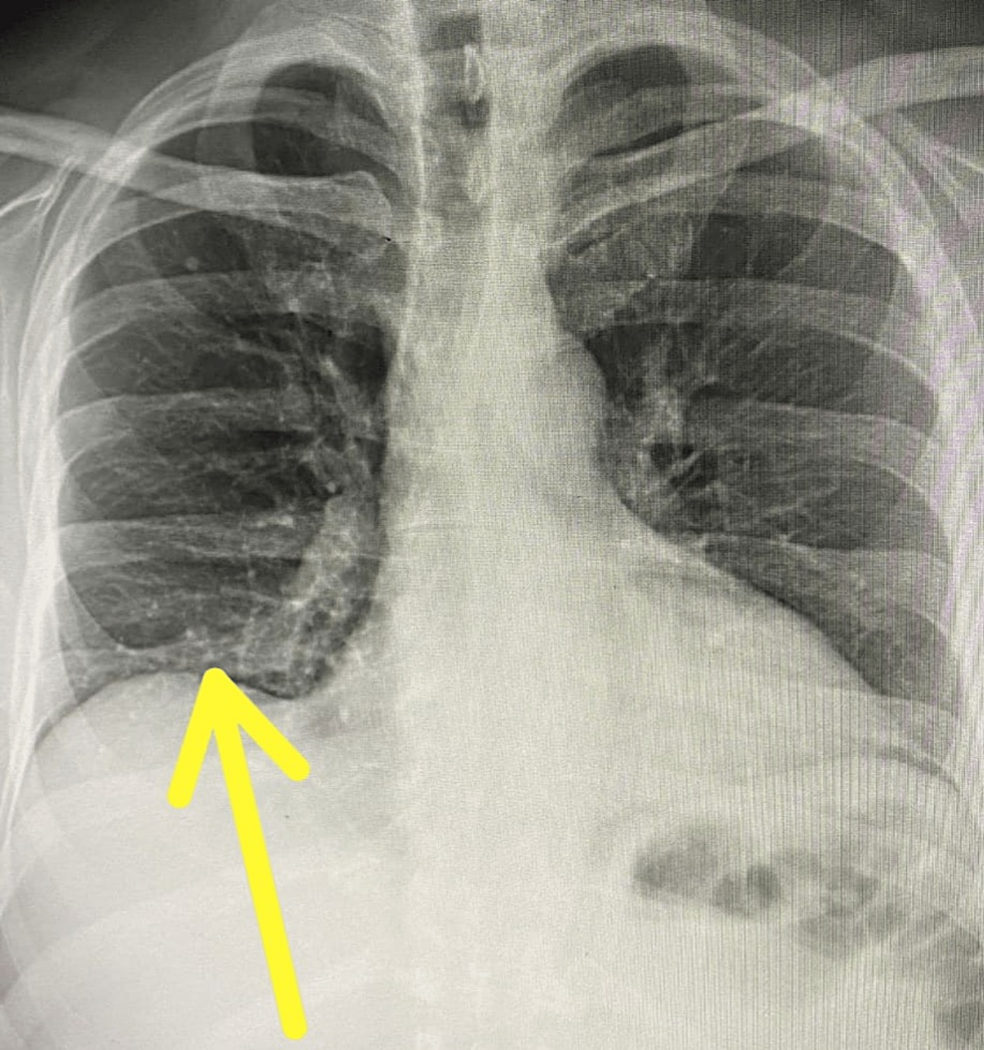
Chest X-ray image on admission The arrow shows pulmonary infiltrates.
